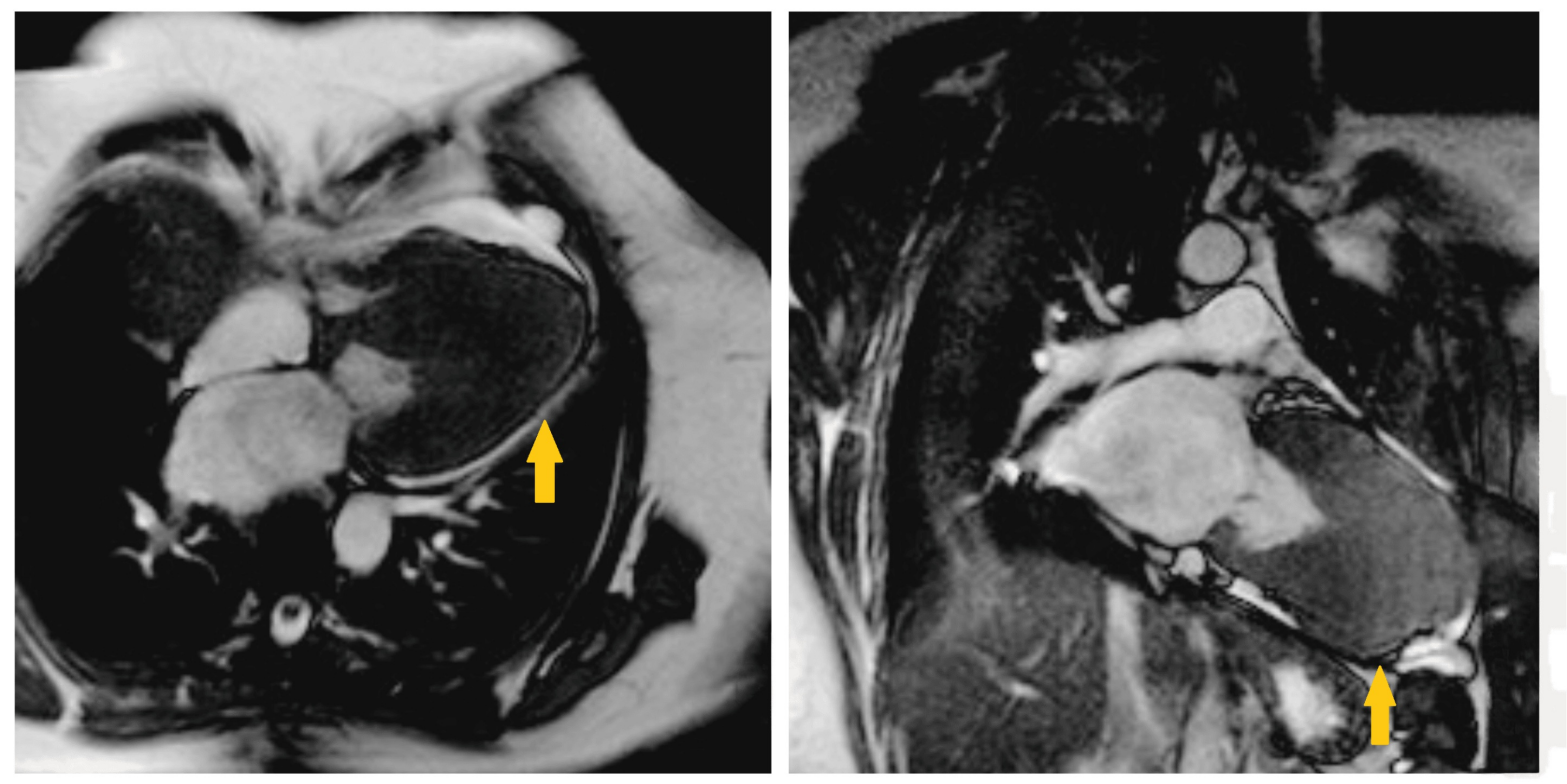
Cardiac MRI showing apical and mid-segment left ventricular hypertrophy (yellow arrows)
